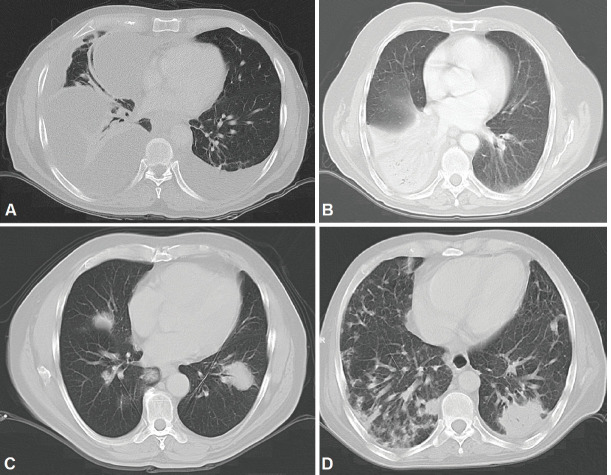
CT scans. A: Bilateral pleural effusion mainly right, loculated pleural effusion B. Consolidation with left lower lobe air bronchogram. C: Consolidation with air bronchogram D: Bilateral multifocal consolidations with air bronchogram.
